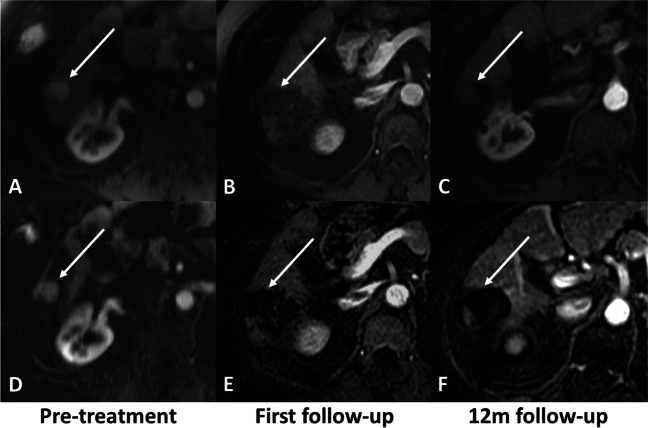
74-year-old female with NASH cirrhosis and right hepatic lobe HCC treated with radiation segmentectomy. MRI obtained during the arterial phase (upper row) and arterial phase subtraction images (lower row). Pre-treatment images show hyperenhancing lesion (arrow in A and D). 1^st^ follow-up MRI post radiation segmentectomy (B and E) demonstrates completely necrotic tumor with no residual enhancing component, and was classified as complete response according to mRECIST, LR-TR, and subtraction by the 3 readers. The treatment response at the 12-month follow-up was categorized as complete response according to mRECIST (C and F)
